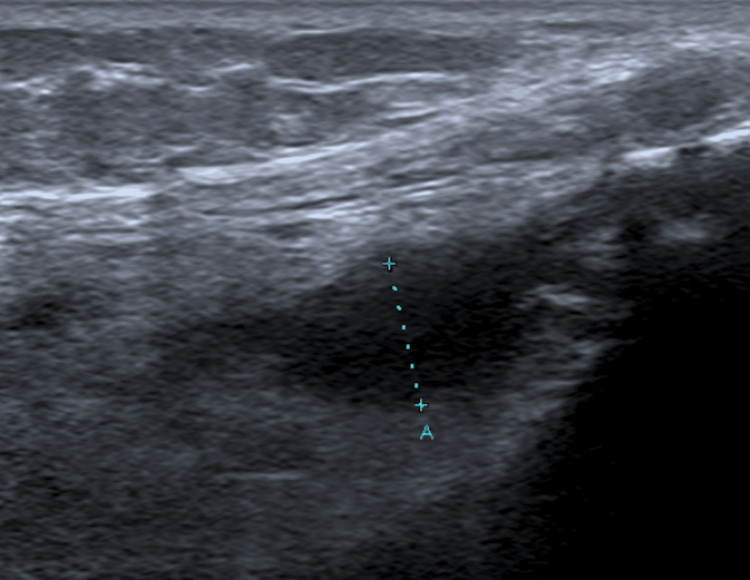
Right knee ultrasound Small-volume joint effusion measuring 5 mm in thickness is observed in the suprapatellar bursa, slightly more pronounced in the external recess of the joint cavity, where it measures 7 mm in thickness.
